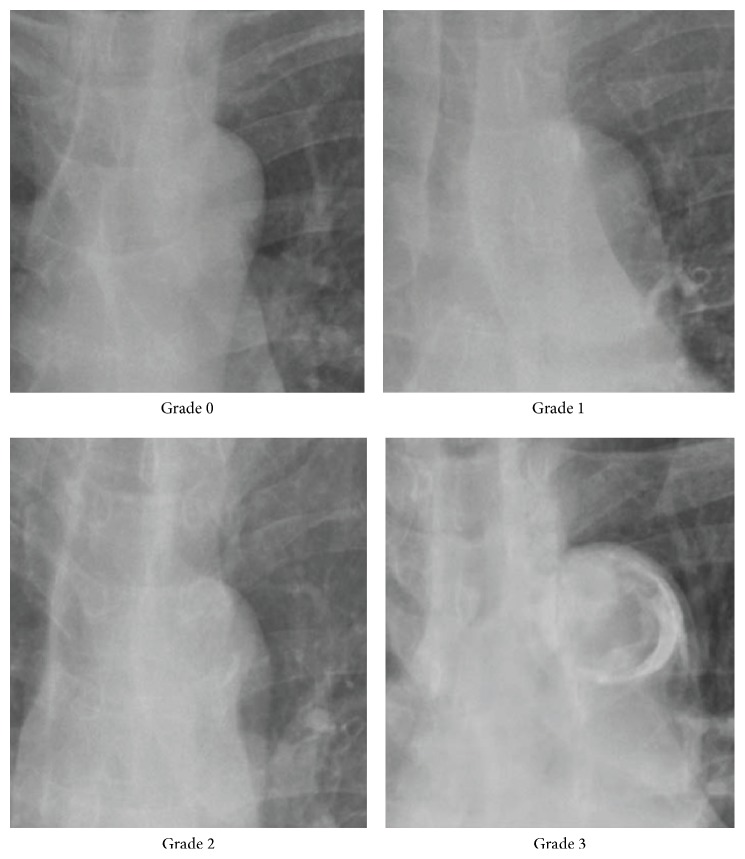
Representative images of aortic arch calcification on postero-anterior chest radiograph are shown. Aortic arch calcification extent is divided into four grades according to the categorization.
